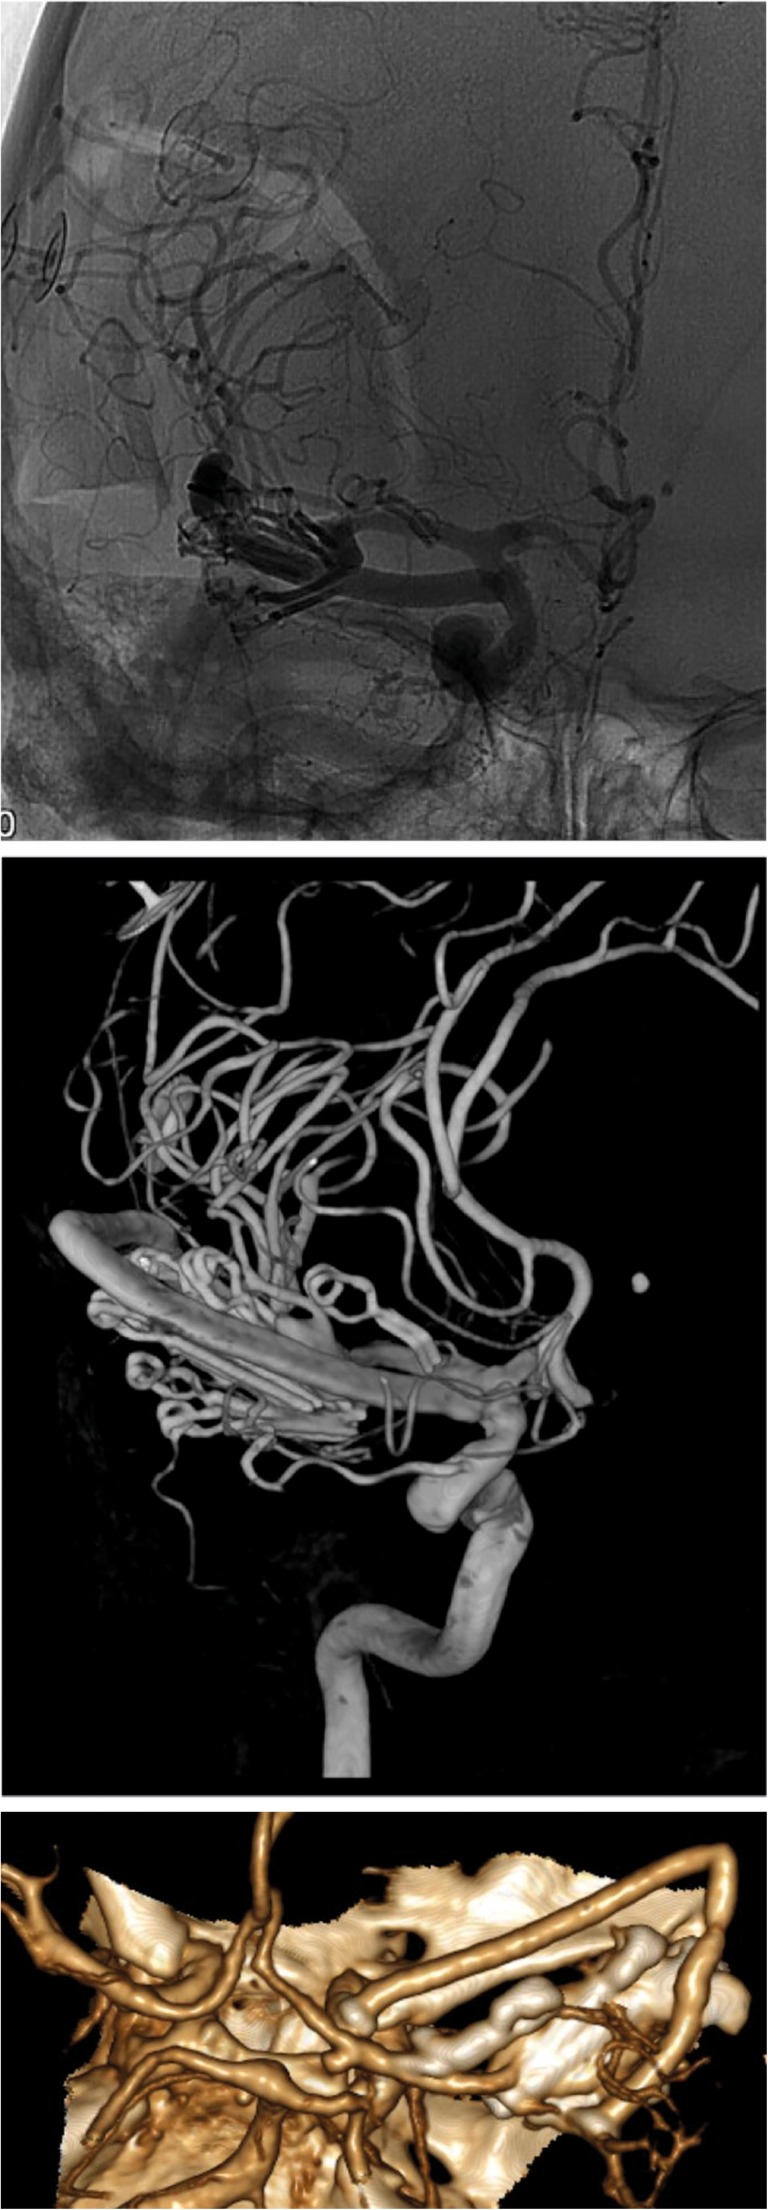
Postoperative imaging of ICA-M2 and IC-IC SELANA slide bypass. DSA (a), 3D angiography (b), and CTA (c) with a confined aneurysm is trapped. Left DSA: #, abandoned first SELANA slide; *, second SELANA slide on the ICA; ^, third SELANA slide on an M2 branch
